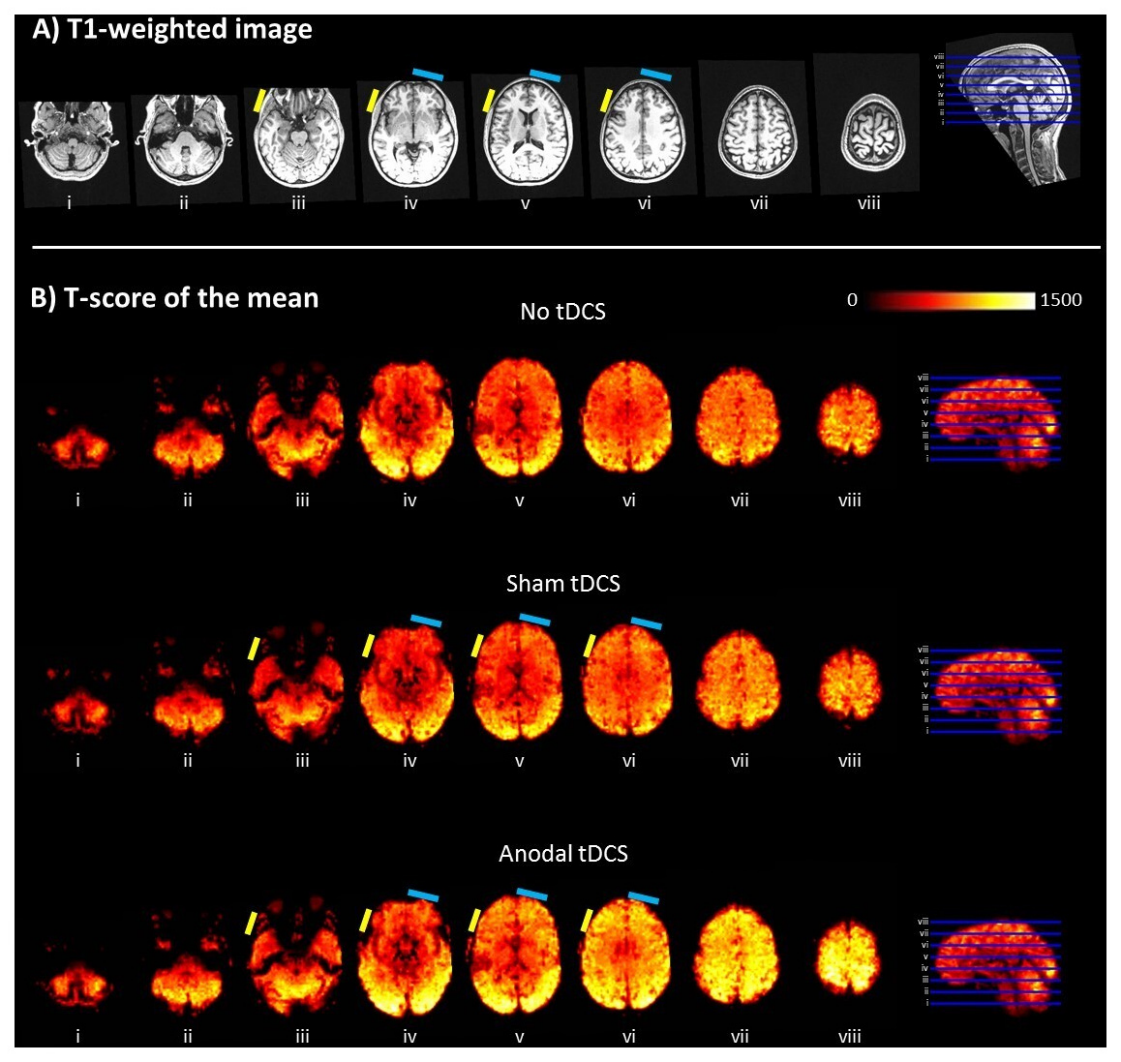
Quality assessment of MR images acquired on one participant. A) High-resolution (1mm, isotropic) structural T1-weighted image denoting electrode locations shown as a reference.
